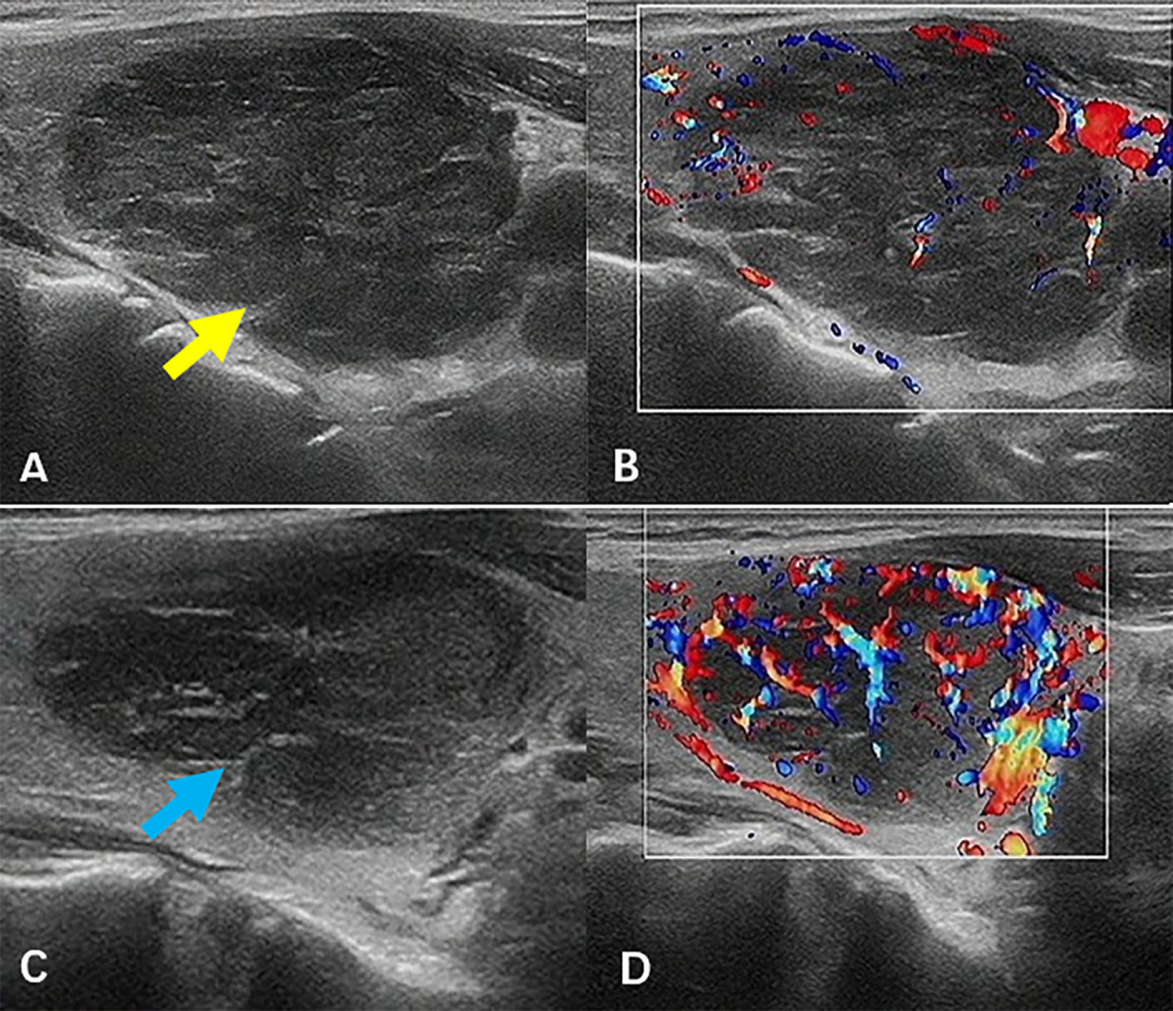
Ultrasound images. Hypoechoic lesions were evident in both thyroid gland lobes (A, B, the left lobe, yellow arrow; C, D, the right lobe, blue arrow), with a clear boundary, a regular shape, and an uneven internal echo. CDFI revealed abundant blood flow signals in and around the lesions. CDFI, color doppler flow imaging.
